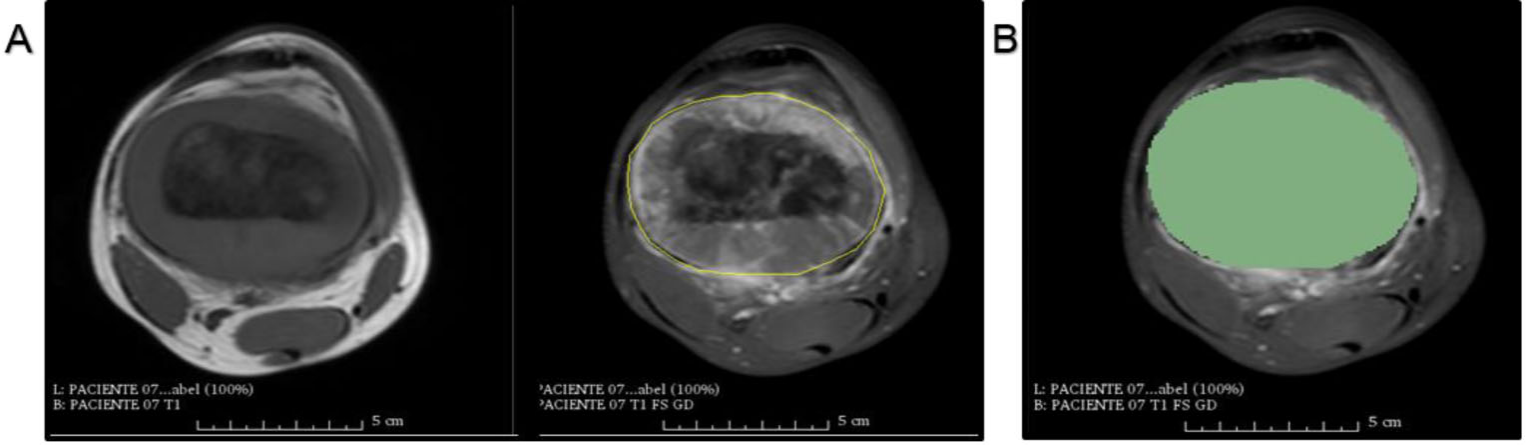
Slicer's GrowCut manual segmentation on axial plane using femoral osteosarcoma magnetic resonance imaging. A, T1 sequence image is on the left and T1 FS GD sequence image is on the right. Manual segmentation of the tumor in axial T1 FS GD images demarcated by yellow line. B, Same axial T1 FS GD image of (A), after marking the segmented area or “label” by the software (in green). T1WI: T1 weighting; T1WI FS GD: T1 weighting with fat suppression after intravenous administration of gadolinium.
